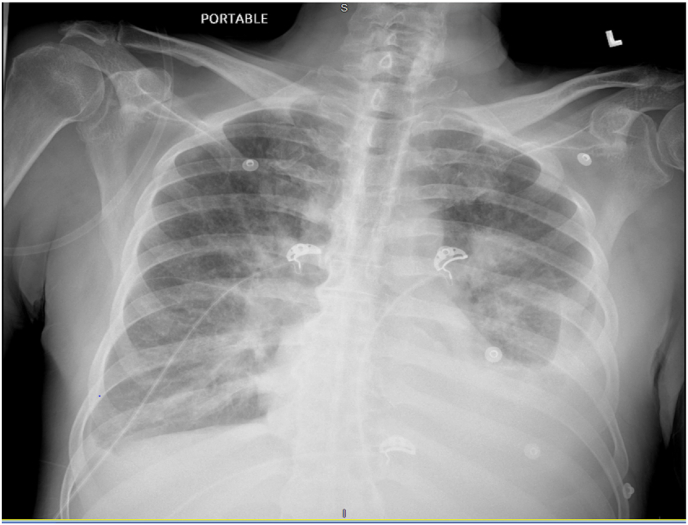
Portable chest radiograph on 4/7/21: Bilateral airspace consolidation with left greater than right pleural effusions.
